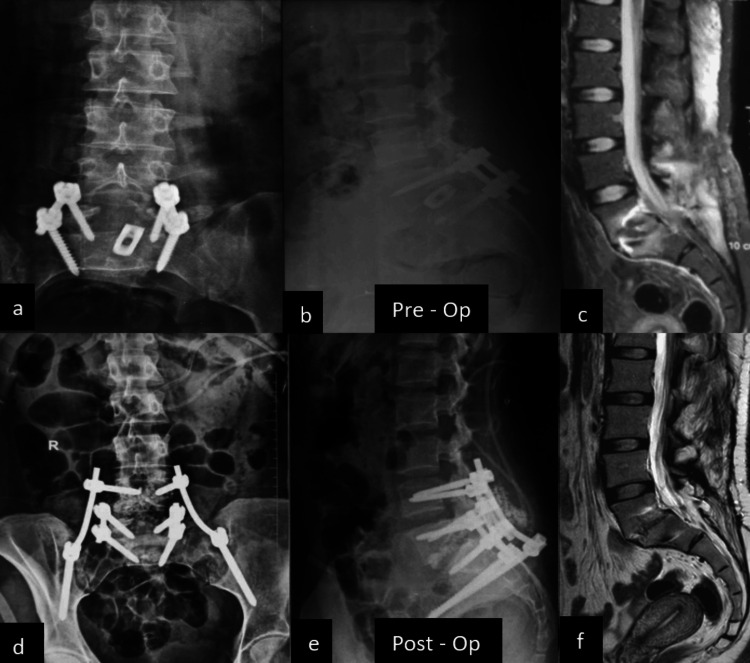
Preoperative and Postoperative Radiological Images of a Patient With L5-S1 Spondylodiscitis With Implant In Situ Operated With Cage Removal and Revision Implantation, Increasing Proximal and Distal Anchorage With Stimulan Insertion (Case 5) (a) Preoperative AP LS spine X-ray; (b) preoperative lateral LS spine X-ray; (c) preoperative sagittal T2W MRI image; (d) immediate postoperative AP LS spine X-ray; (e) immediate postoperative lateral LS spine X-ray; (f) postoperative sagittal T2W MRI image at six months T2W, T2-weighted; LS, lumbosacral
